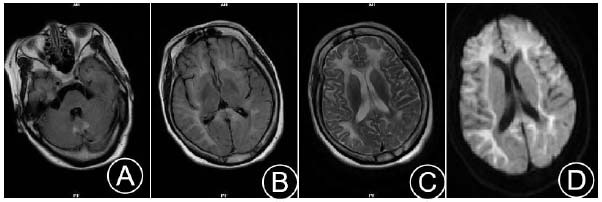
Cranial MRI: Symmetrical small patchy high signals in the cerebellar vermis on T2WI (A); symmetrical large patchy high signals in the corticomedullary junction, centrum semiovale, and the genu and splenium of the corpus callosum on FLAIR and T2WI (B, C); DWI shows band-like high signals in the corticomedullary junction of both cerebral hemispheres, with high signals in the genu and splenium of the corpus callosum (D).
